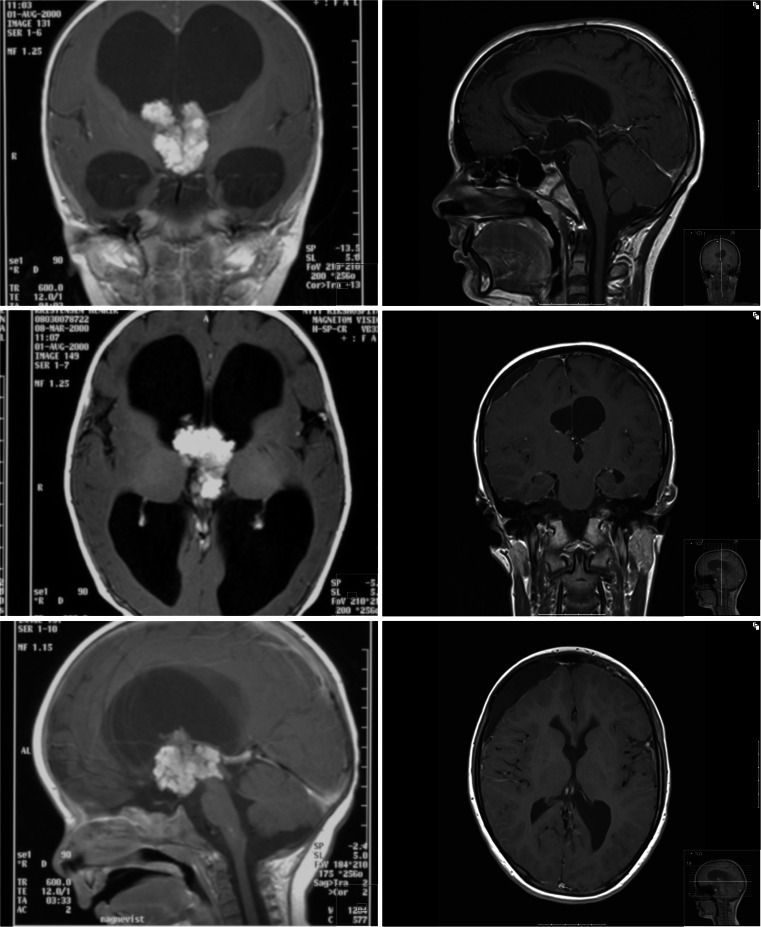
Patient 16. Five-month-old boy presenting with rapidly increasing head circumference and tense fontanelle. Preoperative and postoperative axial and sagittal MRI scans after GTR for the third ventricle plexus papilloma. The patient has 14-year event-free survival
